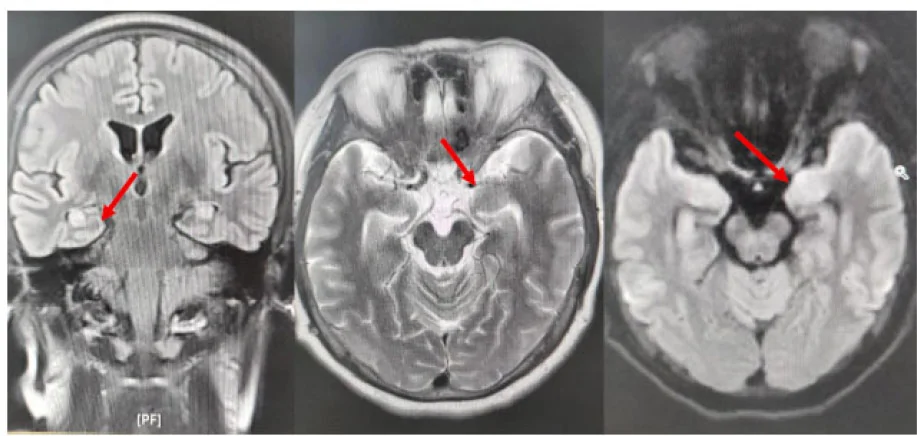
Brain MRI shows bilateral temporal lobe hippocampi with high T2WI signal intensity and blurred margins. FLAIR imaging shows increased signal intensity, more pronounced on the left side.
